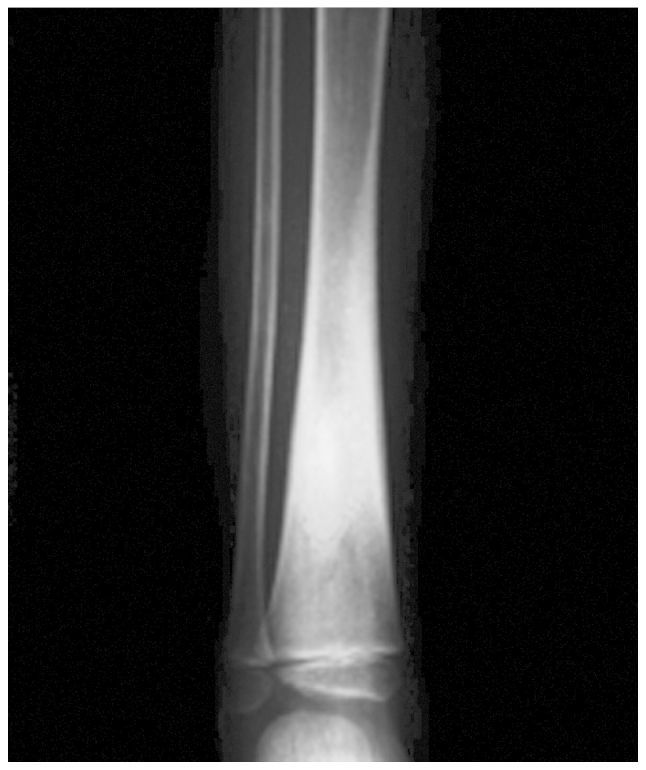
Anteroposterior radiography showed increased radiopaque nidus in the distal tibia.
